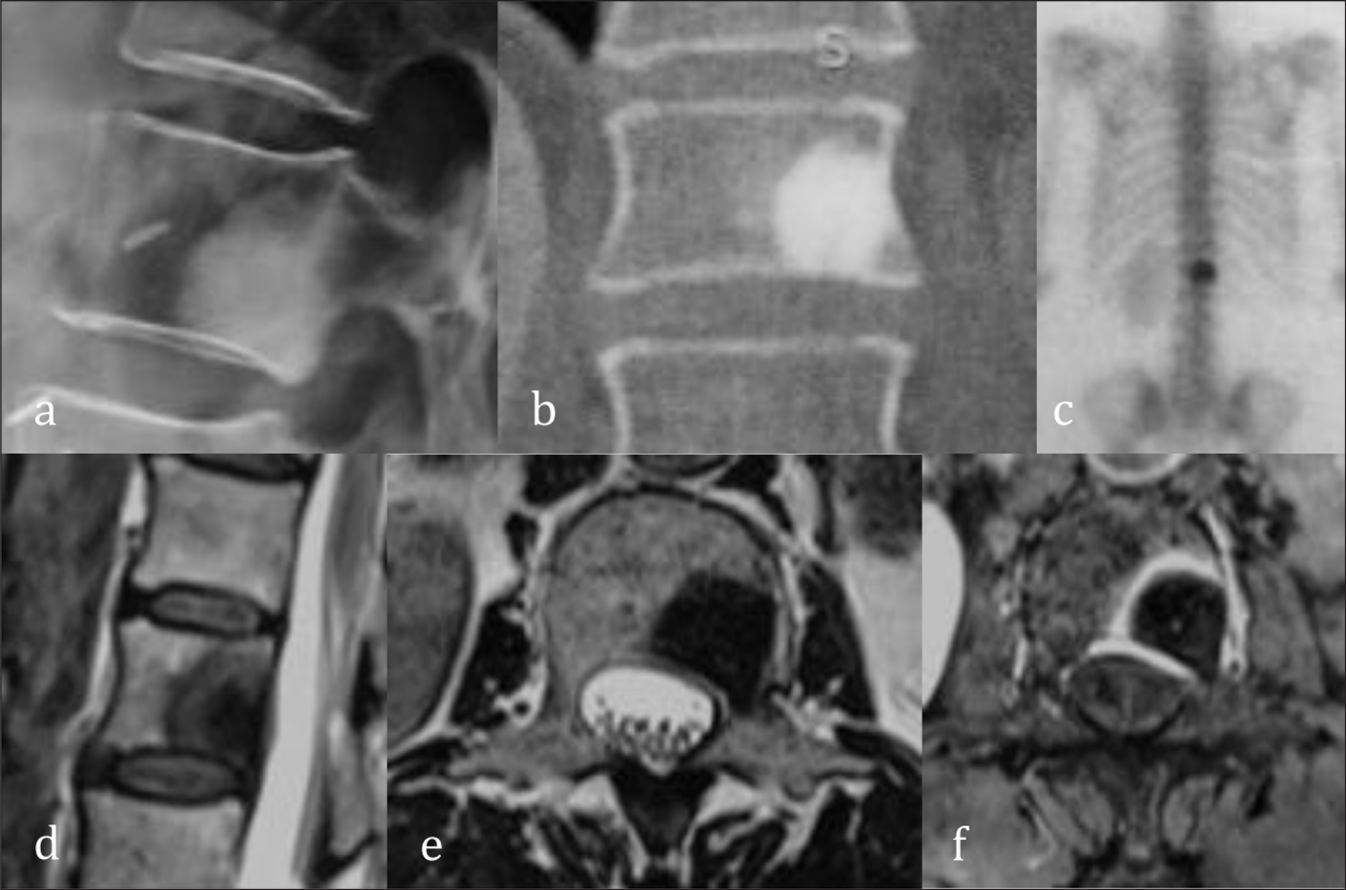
Enostosis of L1. Radiograph (a) and coronal reformatted CT (b) show a focal area of dense bone similar to cortical bone. Bone scintigraphy (c) show an avid uptake. Sagittal T2-WI (d), axial T2-WI (e) and axial T1-WI after administration of gadolinium contrast (f) show central low signal intensity surrounded by intermediate T2 signal and peripheral enhancement.
